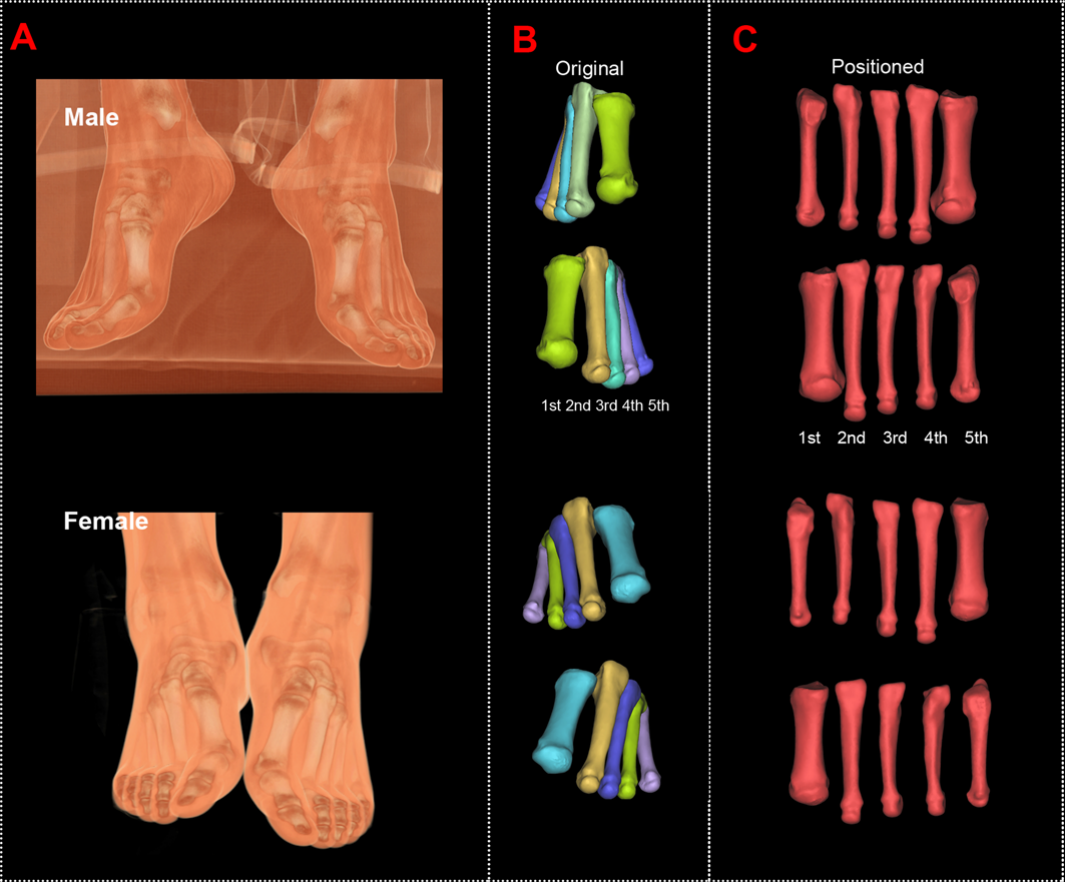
Computed tomography reconstructions of the original and positioned scanning posture of the investigated metatarsal bones. (A) Scanning postures of a male and a female subject. (B) Reconstructed first to fifth metatarsal bones of the male and female subjects. (C) Positioned first to fifth metatarsal bones of the male and female subjects.
